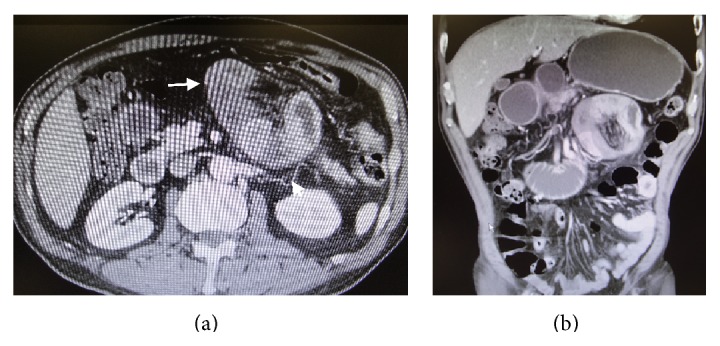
An axial computed tomography scan shows an encapsulated cluster of dilated small bowel loops (arrow) occupying the upper quadrant. A small bowel loop is ascending the left colic artery (arrowhead).
